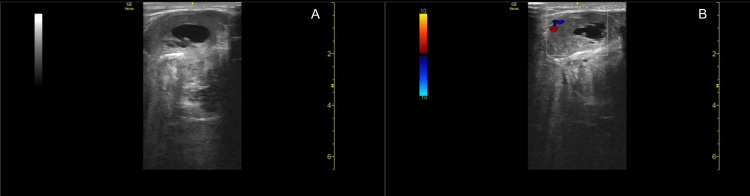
Bedside ultrasound. Two views of the initial bedside ultrasound of the right posterior thigh demonstrating a heterogeneous mass with a hypoechoic center (A) with questionable vascular bundle invasion on the Doppler setting (B).
